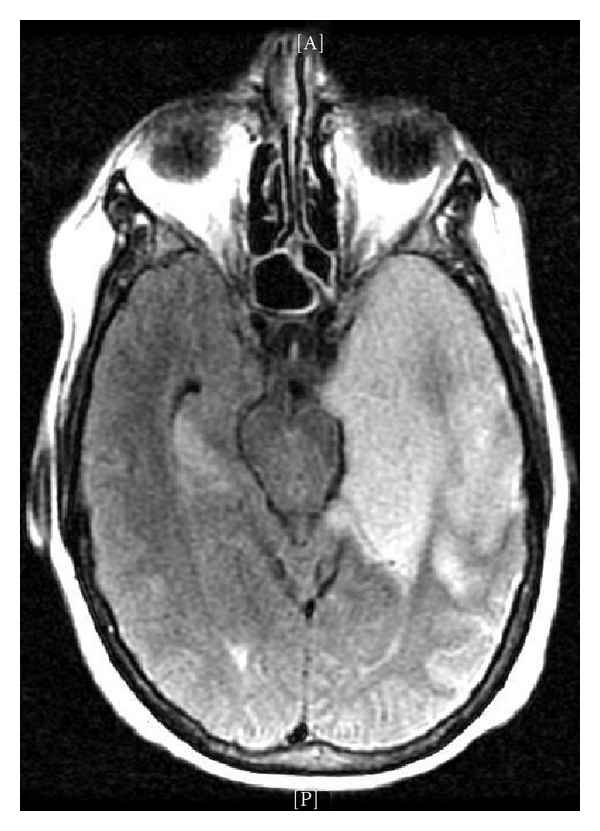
MRI T2 FLAIR: hyperintensity of lesions on T2.
